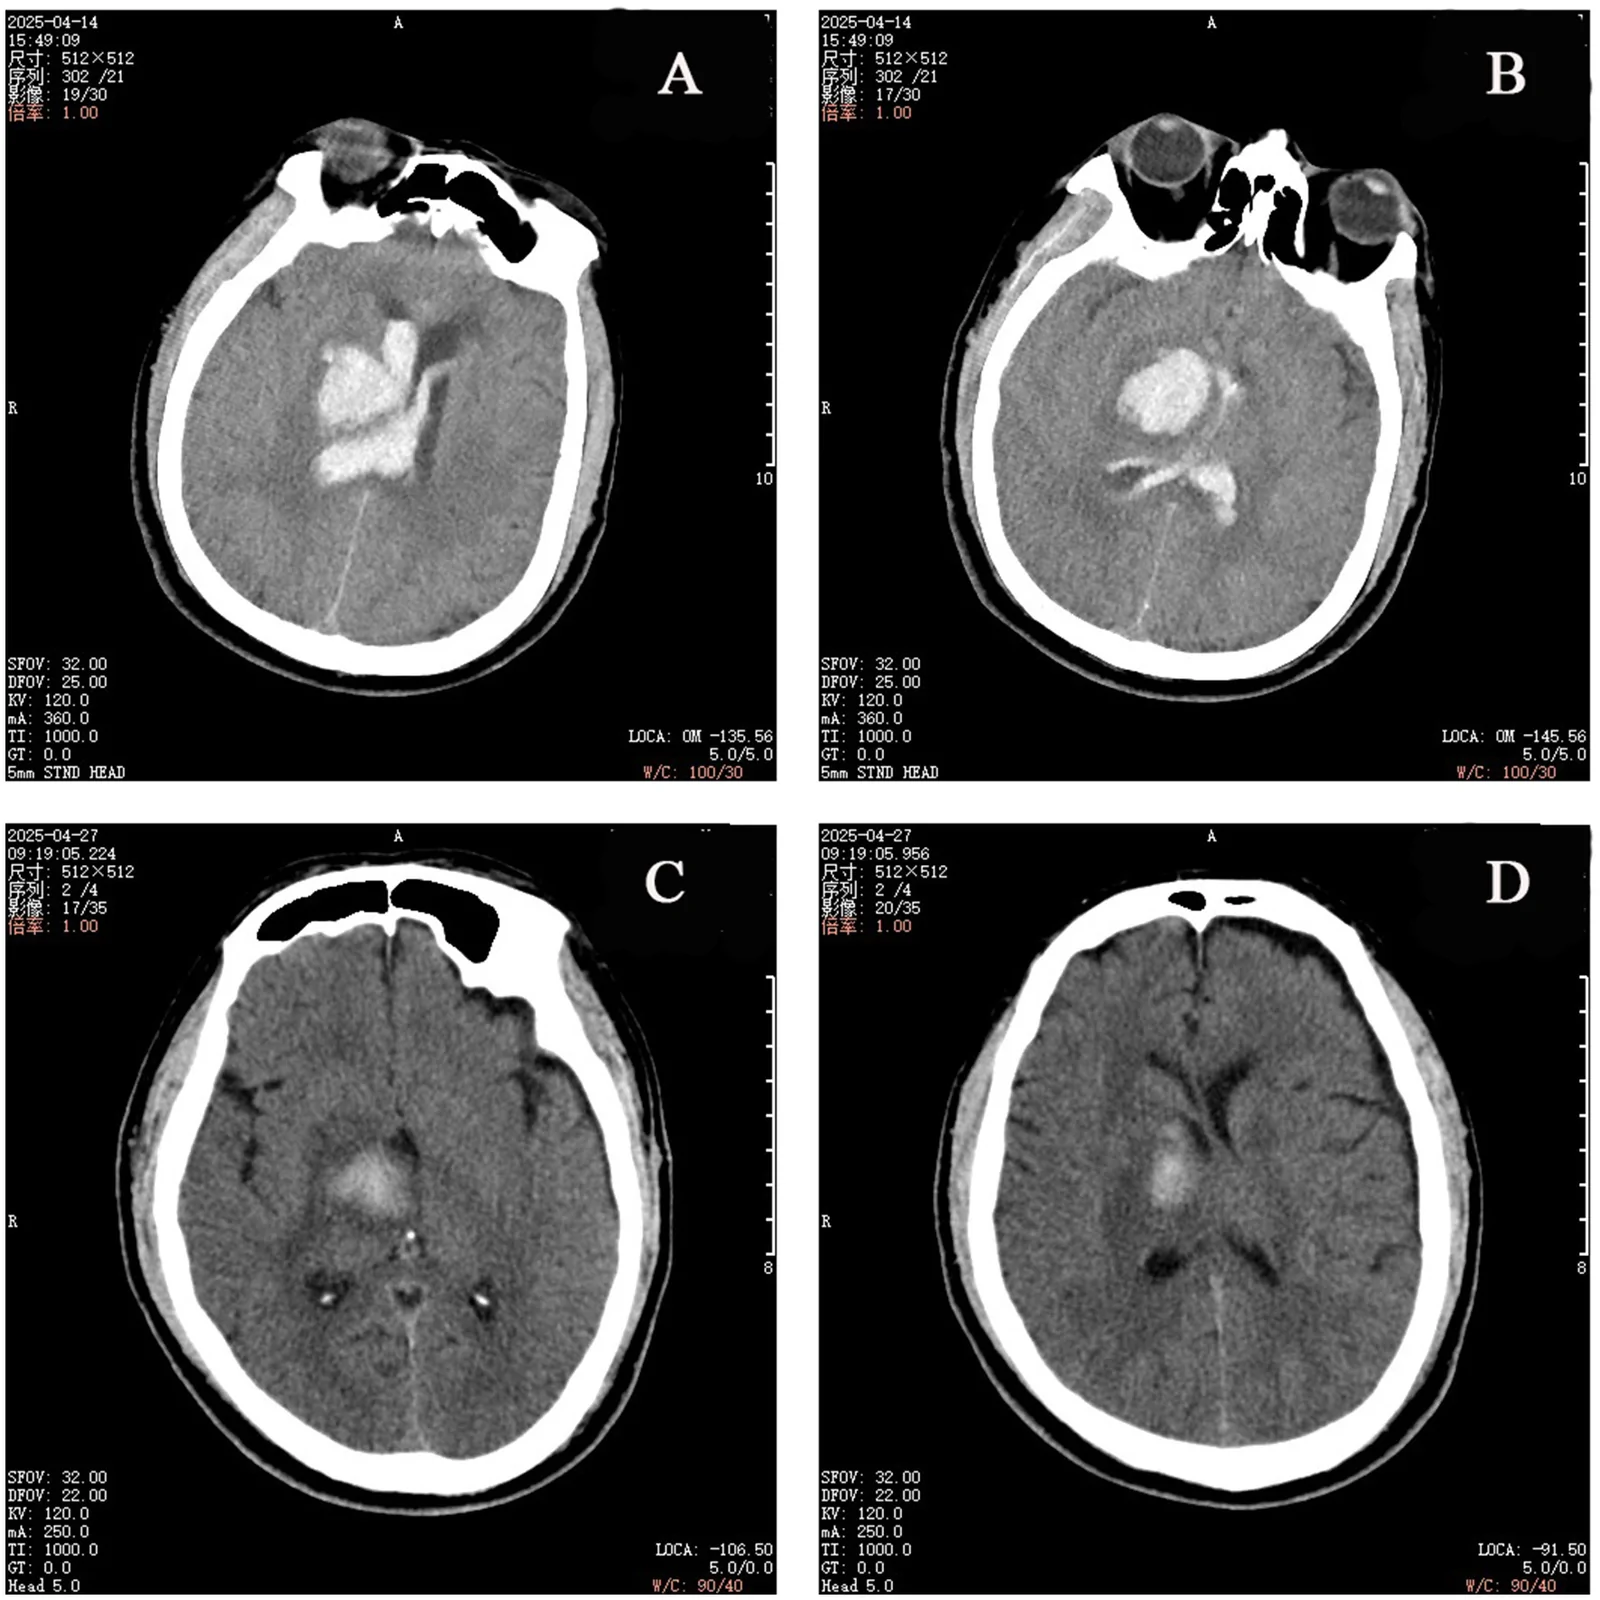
Preoperative and postoperative cranial CT images of Patient 4 with massive intraventricular hemorrhage. (A, B) Preoperative CT scans demonstrate massive intracerebral hemorrhage with ventricular extension and significant cerebral swelling. (C, D) Postoperative CT scans following hematoma evacuation and external ventricular drainage show successful removal of the hematoma and marked improvement of cerebral edema.
